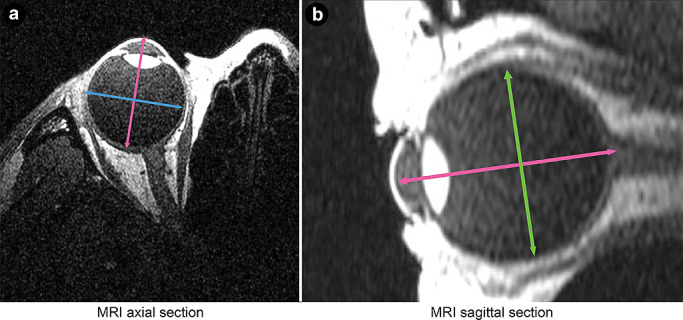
MRI images of (a) an axial section and (b) a sagittal section The AL, shown by red lines in both the axial and sagittal planes, is the distance from the corneal anterior surface to the approximate position of the fovea centralis. The HD, shown by the green line, is the maximum diameter across the retina of a horizontal line that intersects the eye axis on the axial plane image. VD is the maximum diameter across the retina of a vertical line that intersects the eye axis on the sagittal plane image. MRI, magnetic resonance imaging; AL, axial length; HD, horizontal distance; VD, vertical distance
